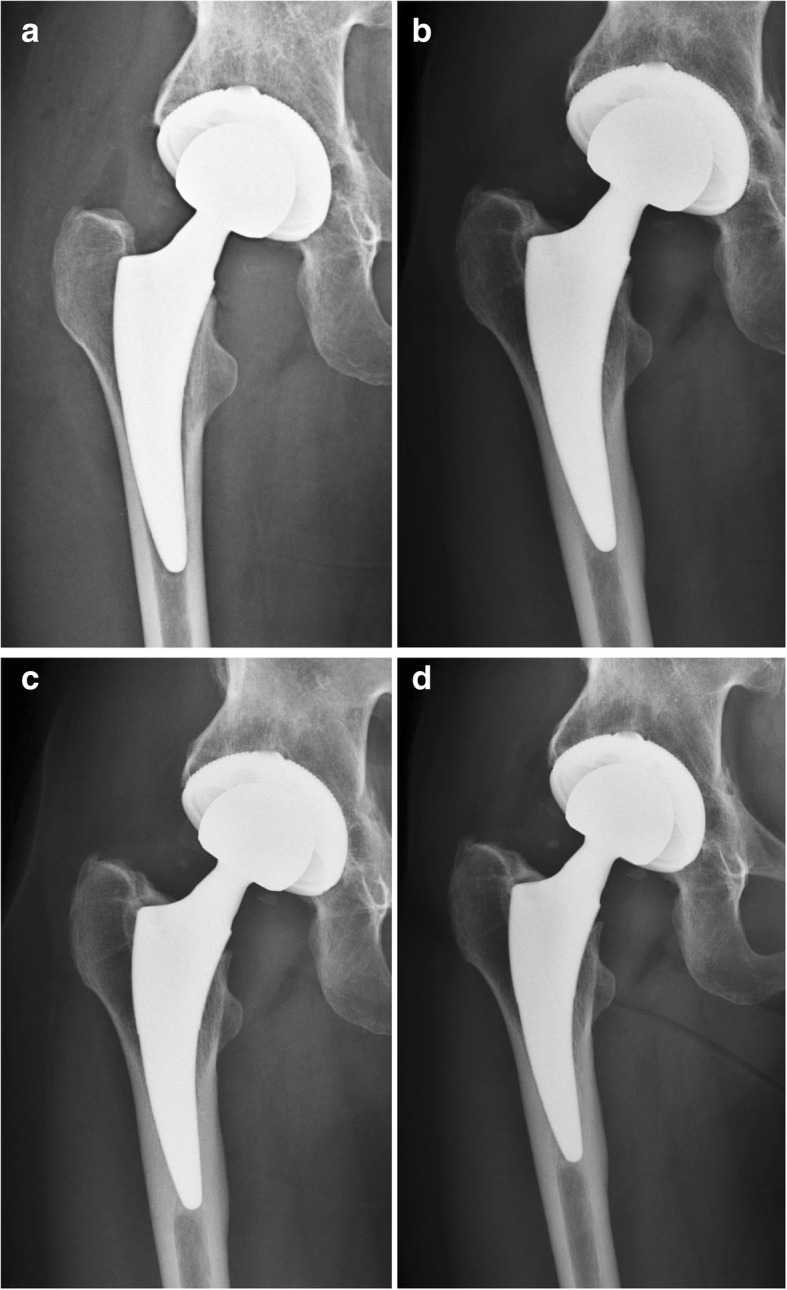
Radiographs of a 50-year-old female patient with a typical course of a short femoral Fitmore hip stem. a immediately after surgery with radiolucencies at the lesser trochanter, b at 1 year postoperatively showing cortical hypertrophy in Gruen zones 3, 5 and 6, and a decrease in radiolucencies, but also cortical thinning around the lesser trochanter, c at 2 years with equal findings, d after 5 years with cortical hypertrophy, disappearance of radiolucencies, but with cortical thinning at the level of the lesser trochanter
